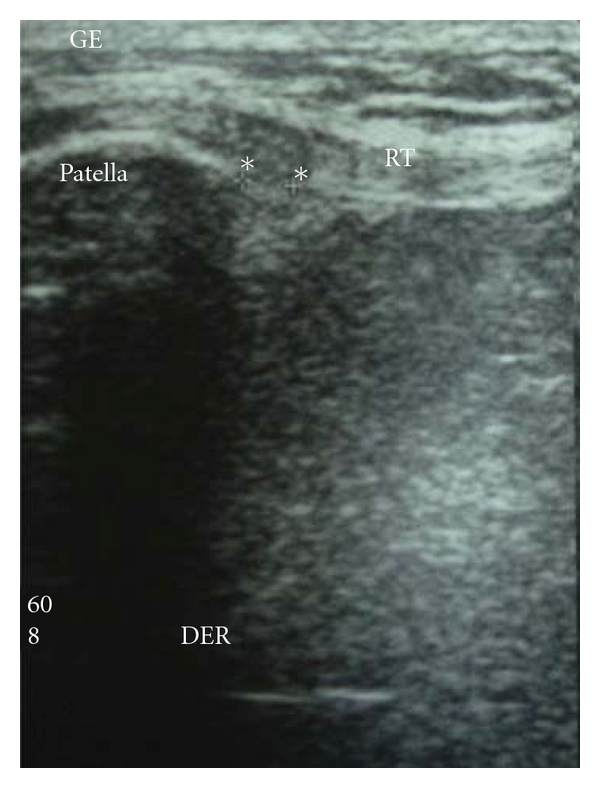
Preoperative US (ultrasound) of the patellar tendon showing areas of edema (hypoechoic) (). RT: rotulian or patellar tendon.
